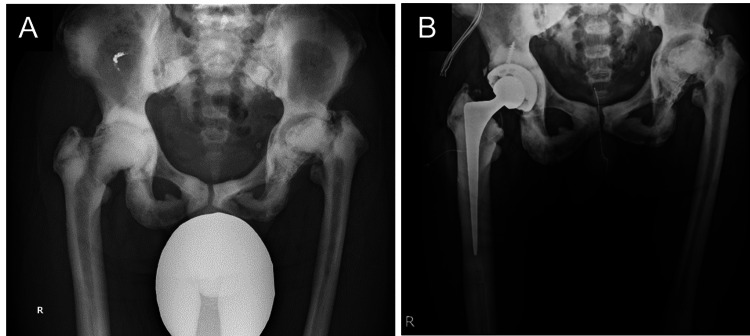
X-ray images of the hip A, preoperative X-ray image; B, postoperative X-ray image Hip osteoarthritis associated with osteopetrosis was observed in both sides.
